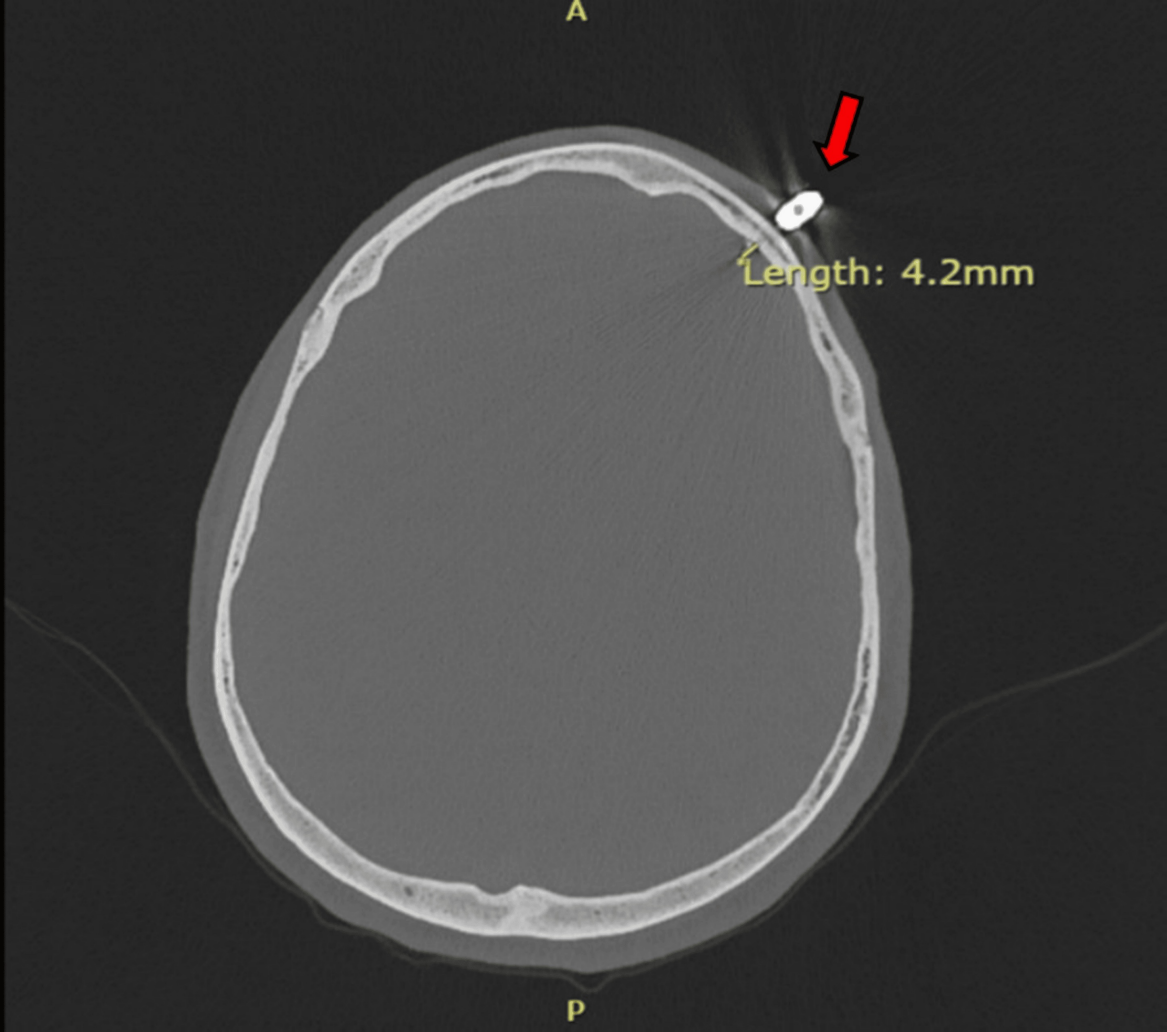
Computed tomography angiogram of the head with and without contrast displaying metallic foreign body embedded within the left frontal cranium (red arrow) and extending 4 millimeters intracranially, beyond the inner table
